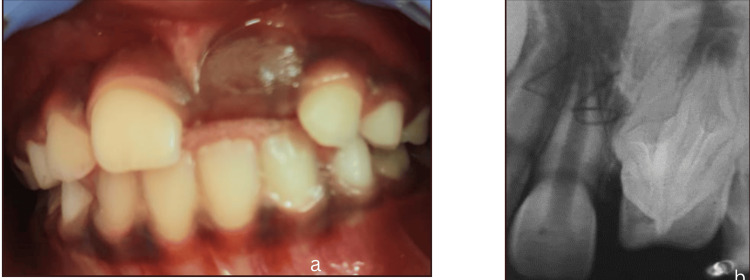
(a) Clinical photograph of the maxillary anterior region revealing unerupted 21 after eight years following trauma. (b) Intra-oral periapical radiograph showing gross malformation of crown and root of unerupted 21.
